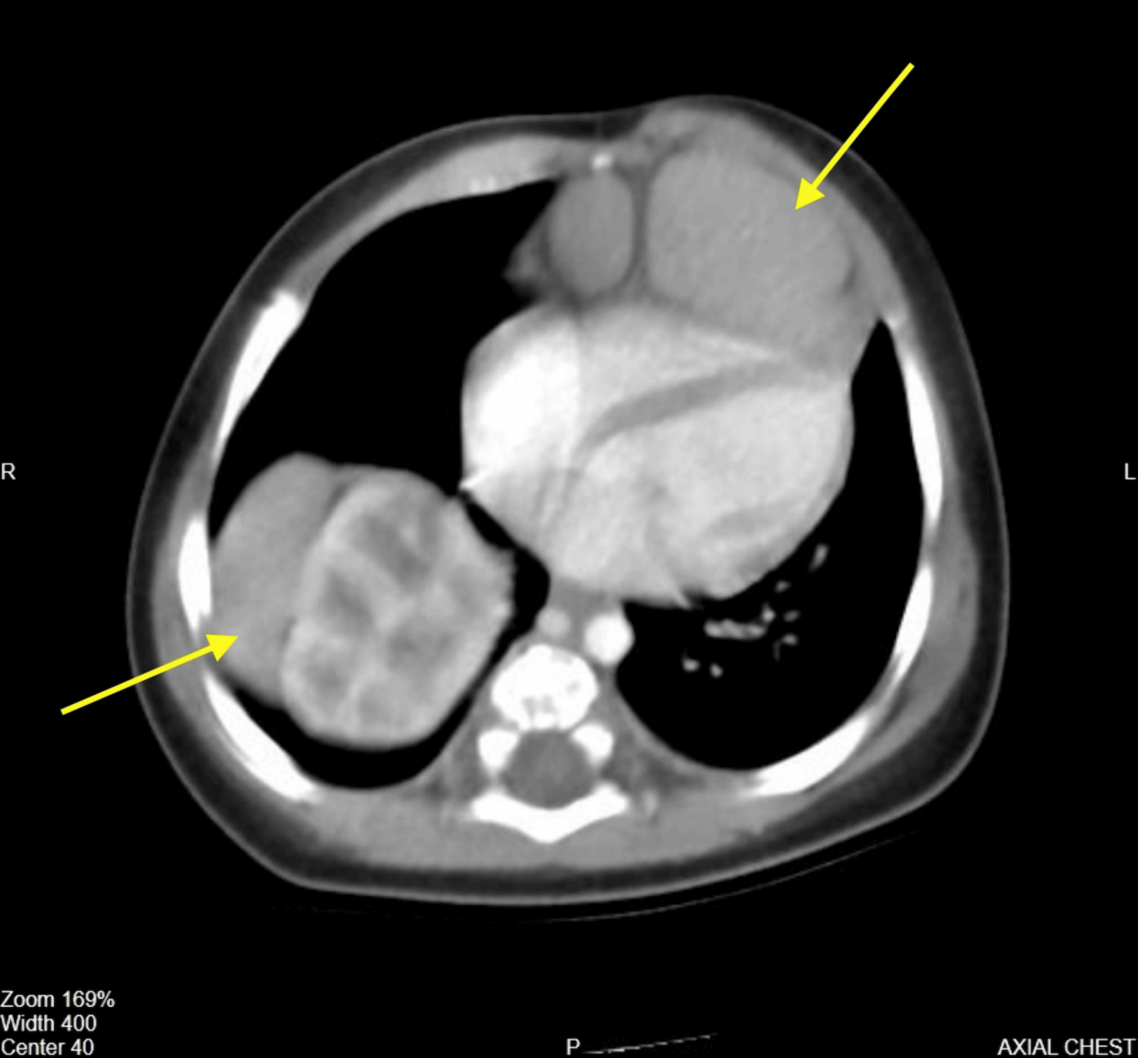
Axial view of the chest CT showing abdominal organs herniated into the thoracic cavity (yellow arrows)
